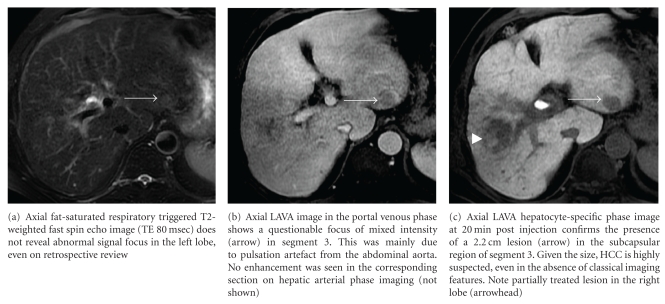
Chronic HBV patient with known multifocal HCC presumed to be confined to the right lobe, completed one session of TACE. US suggested possible nodule in the left hepatic lobe, but this was occult on multiphasic CT. MRI with standard dose of Gd-EOB-DTPA was performed.
